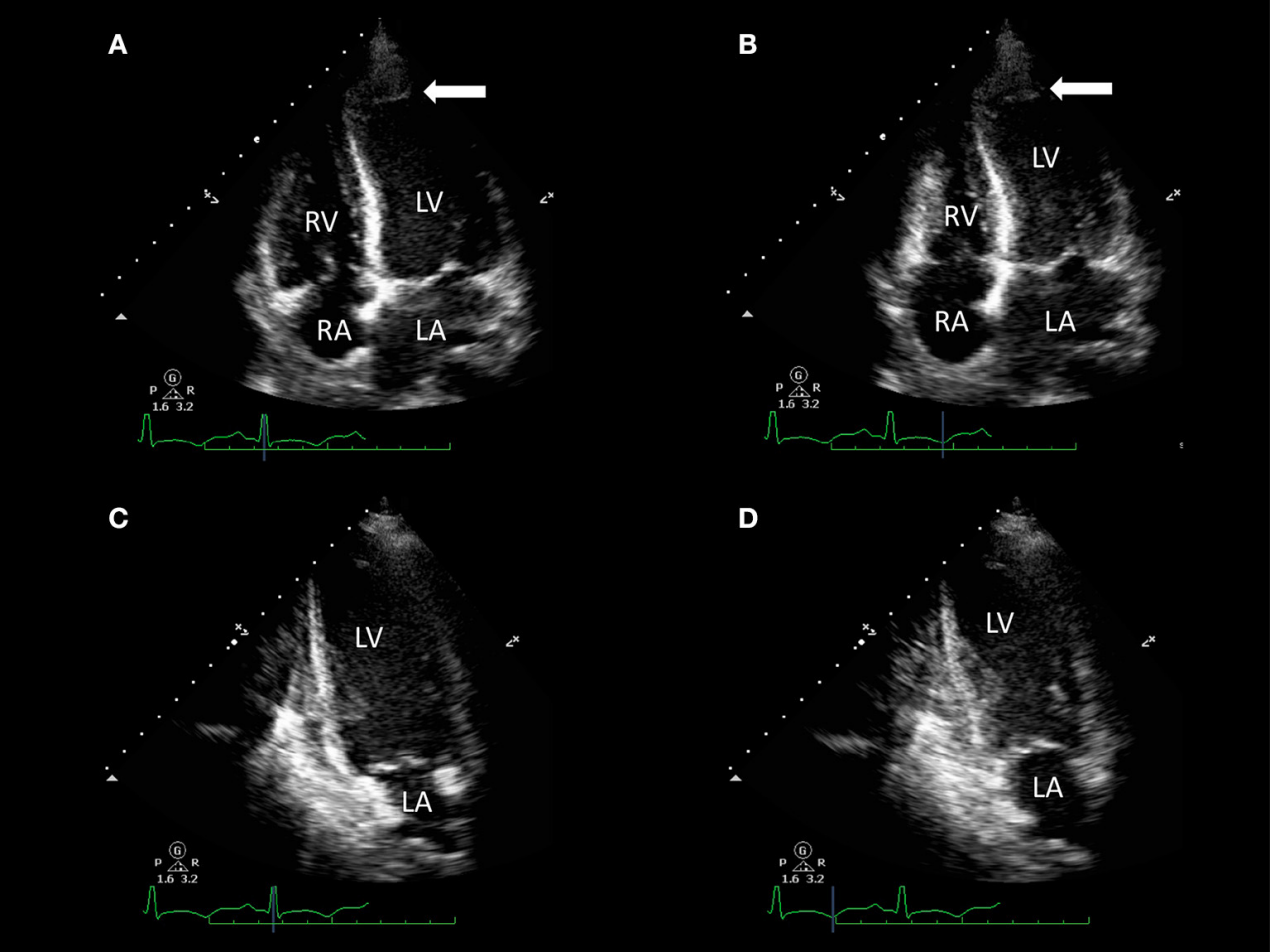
Transthoracic echocardiography of a 32-year-old woman with acute dyspnea 1 day after delivery. Apical four-chamber view [end-diastolic (A) and end-systolic (B) frames] and two-chamber view [end-diastolic (C) and end-systolic (D) frames] show akinesia of apical segments of the left ventricle (LV) and mild-to-moderate LV systolic impairment [LV ejection fraction (LVEF) 45%] with evidence of apical LV thrombus (arrows). Resting right ventricle (RV) function was preserved (fractional area change 48%).
